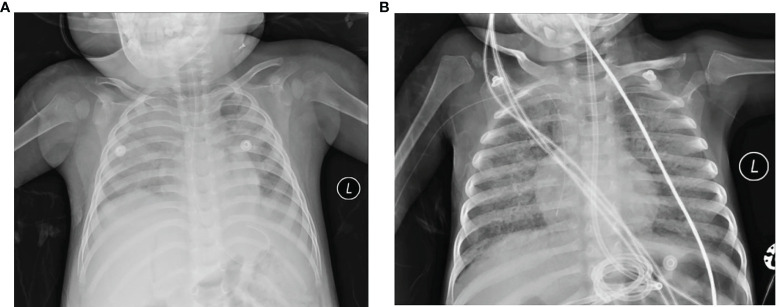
Chest X-ray of the lungs from the acute phage of the two fatal cases. (A) Case 1. Acute phage of pneumonia revealed the texture of the two lungs as thickened and fuzzy, the light transmittance of the left lung is diffusely reduced, left diaphragm blurred, the light transmittance of the right lung unevenly reduced, patchy shadows shown in the right lung. (B) Case 2. The lung light transmittance was unevenly reduced, and multiple high-density shadows and small air bubble emphysema were seen in both lungs. Part of the bronchiectasis and part of the cavity were irregular, especially in the middle lobe of the right lung.
